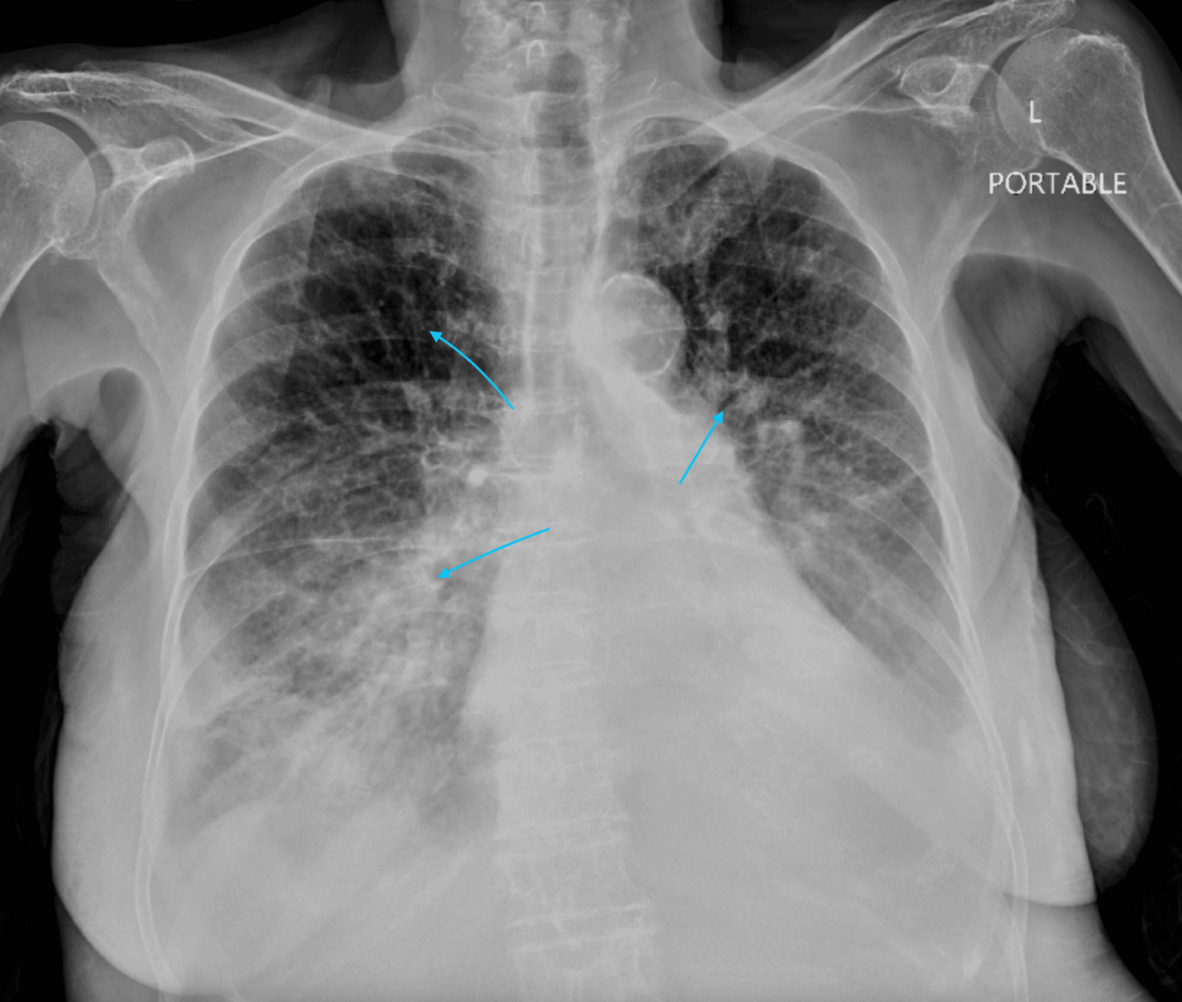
Portable chest X-ray demonstrating pulmonary vascular cephalization, bilateral perihilar congestion, and diffuse interstitial and alveolar opacities consistent with pulmonary edema in the setting of decompensated congestive heart failure
